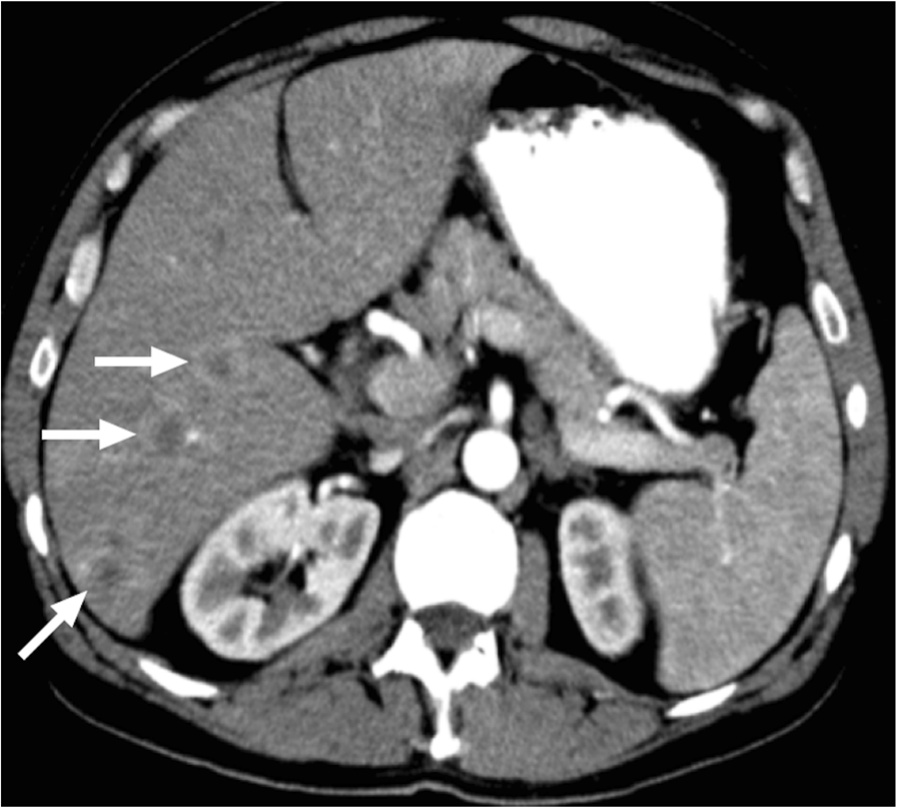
A 48-year-old male with known HIV presenting with recent onset of abdominal pain and mild weight loss. Axial plane postcontrast arterial phase CT image demonstrates several peripherally enhancing liver lesions (arrows) located within the right lobe. Metastatic liver disease or pyogenic microabscesses were initially considered. Percutaneous biopsy was consistent with syphilitic liver abscesses. The RPR test was also found to be positive after the biopsy diagnosis
